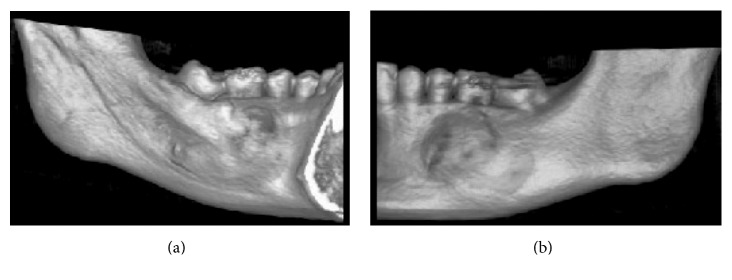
CBCT image, 3D reconstructions. Observe the body of the mandible in the region of the left permanent mandibular first molar. Internal (lingual) view (a) and external (vestibular) view (b).
